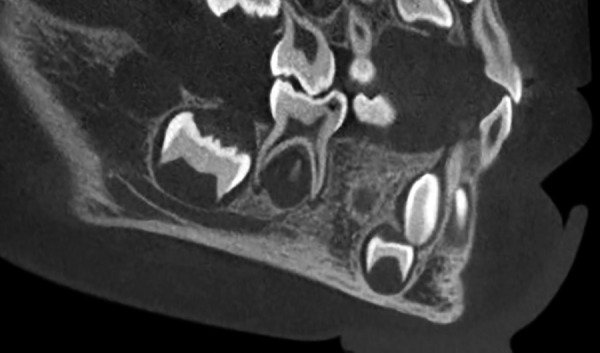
Computed tomography scan. Note: A radiolucent area in the crown extending from the occlusal surface into the dentin under the MB cusp
